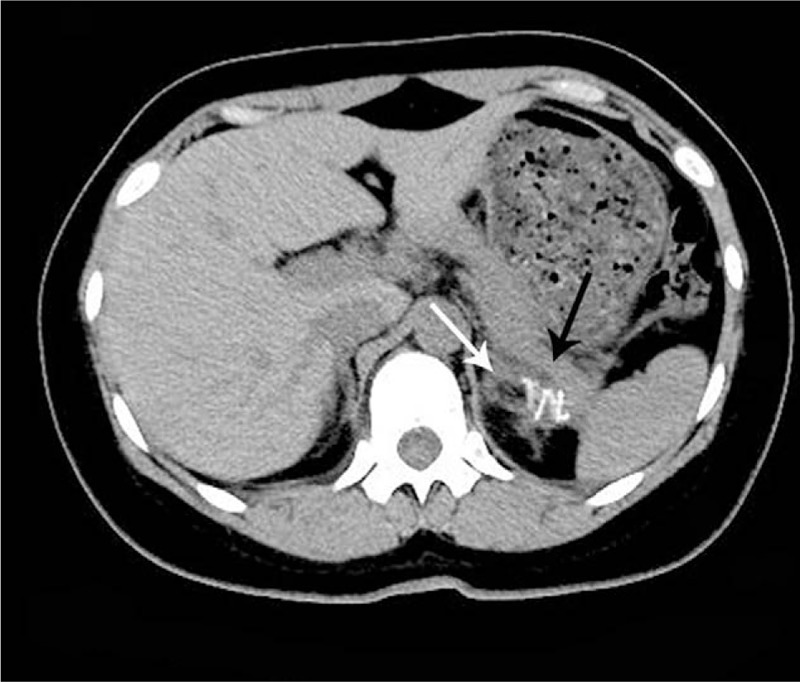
Computed tomography revealed no cystic structure in lesion area, with a relatively normal morphology of adrenal gland (white arrow) and pancreas (black arrows).
